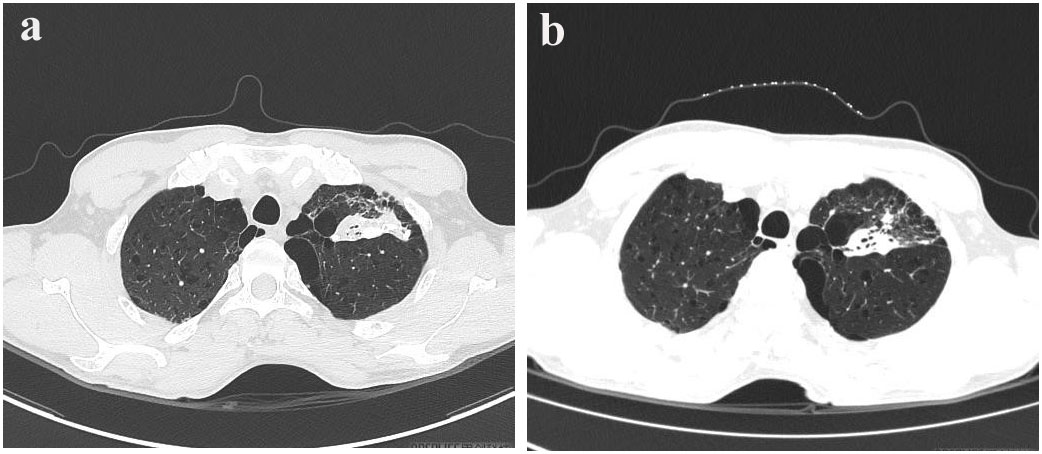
Pre- and post-treatment CT scans in pulmonary tuberculosis. (a) Chest CT scan on January 22, 2025. (b) Follow-up chest CT scan on March 26, 2025.
